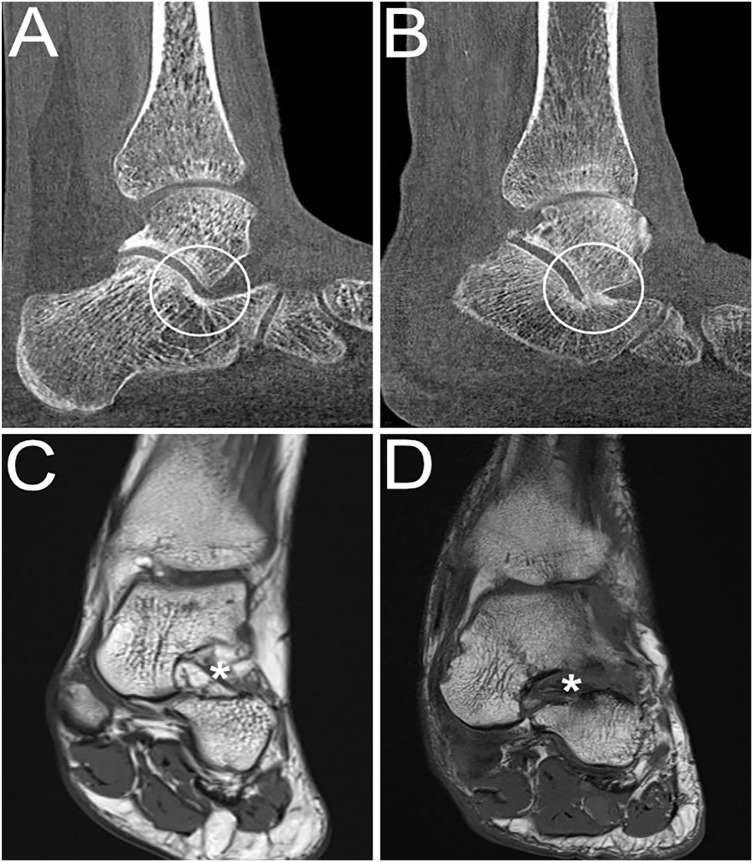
Weightbearing (WB) CT and MR images showing the sinus tarsi in PCFD patients. (A) Sinus tarsi without impingement and (B) sinus tarsi impingement visible on a sagittal WBCT image. (C) T1-weighted coronal MR image showing a normal sinus tarsi (*) and (D) T1-weighted coronal MR image showing complete sinus tarsi obliteration (*) with loss of physiological fat signal. CT, computed tomography; MR, magnetic resonance; PCFD, progressive collapsing foot deformity.
